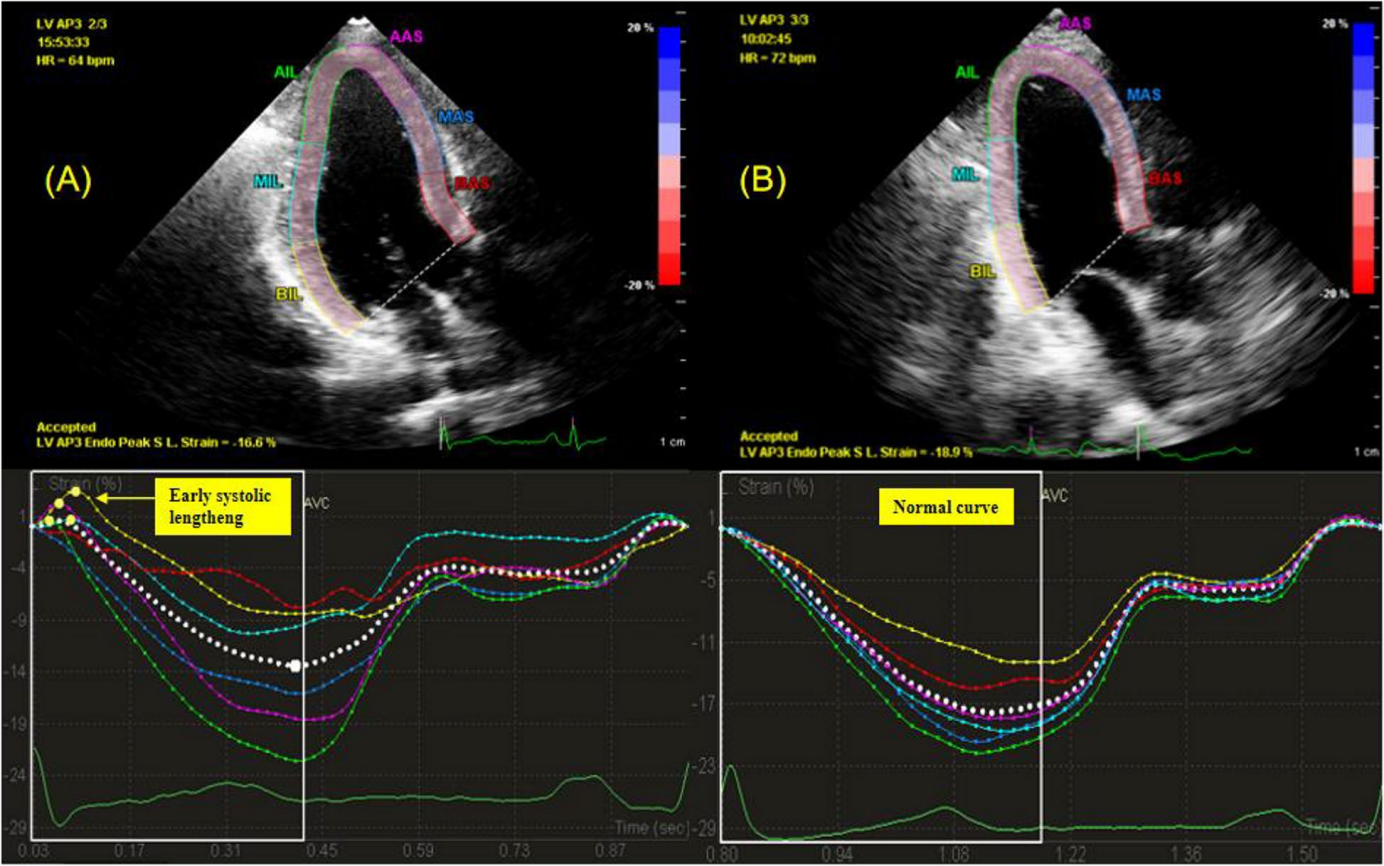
Left ventricular longitudinal strain profiles by speckle tracking echocardiography (STE). Representative image of a non-ST-segment elevation acute coronary syndrome (NSTE-ACS) patient with significant coronary stenosis and myocardial early systolic lengthening (ESL) (A); Representative image of a subject without coronary artery disease (CAD) showing no ESL (B)
